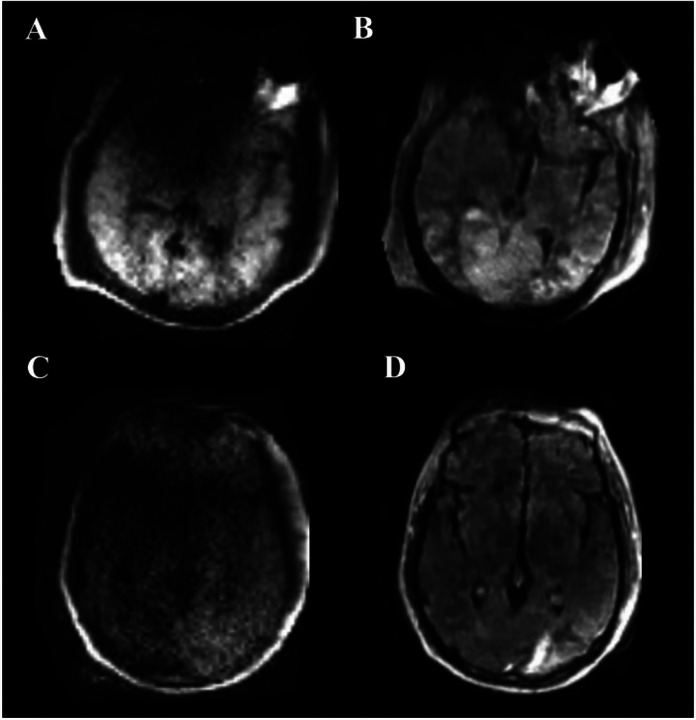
ULF-pMRI images showing artifacts. Overall, DWI MRI (Images A and C) depict artifacts with greater clarity, while FLAIR MRI (B andD) shows fewer artifacts, relatively. Patient 1 (A-B) had an ischemic stroke in the left temporal and both occipital lobes, and Patient 2 (C-D) in the left occipital lobe. Patient 1 has a regional minor artifact anteriorly, obscuring anterior inferior brain, but it does not impact the diagnosis. Patient 2 has a major artifact in the area of the right cerebral hemisphere in general, leading to uncertainty about whether it represents infarct or artifact.
